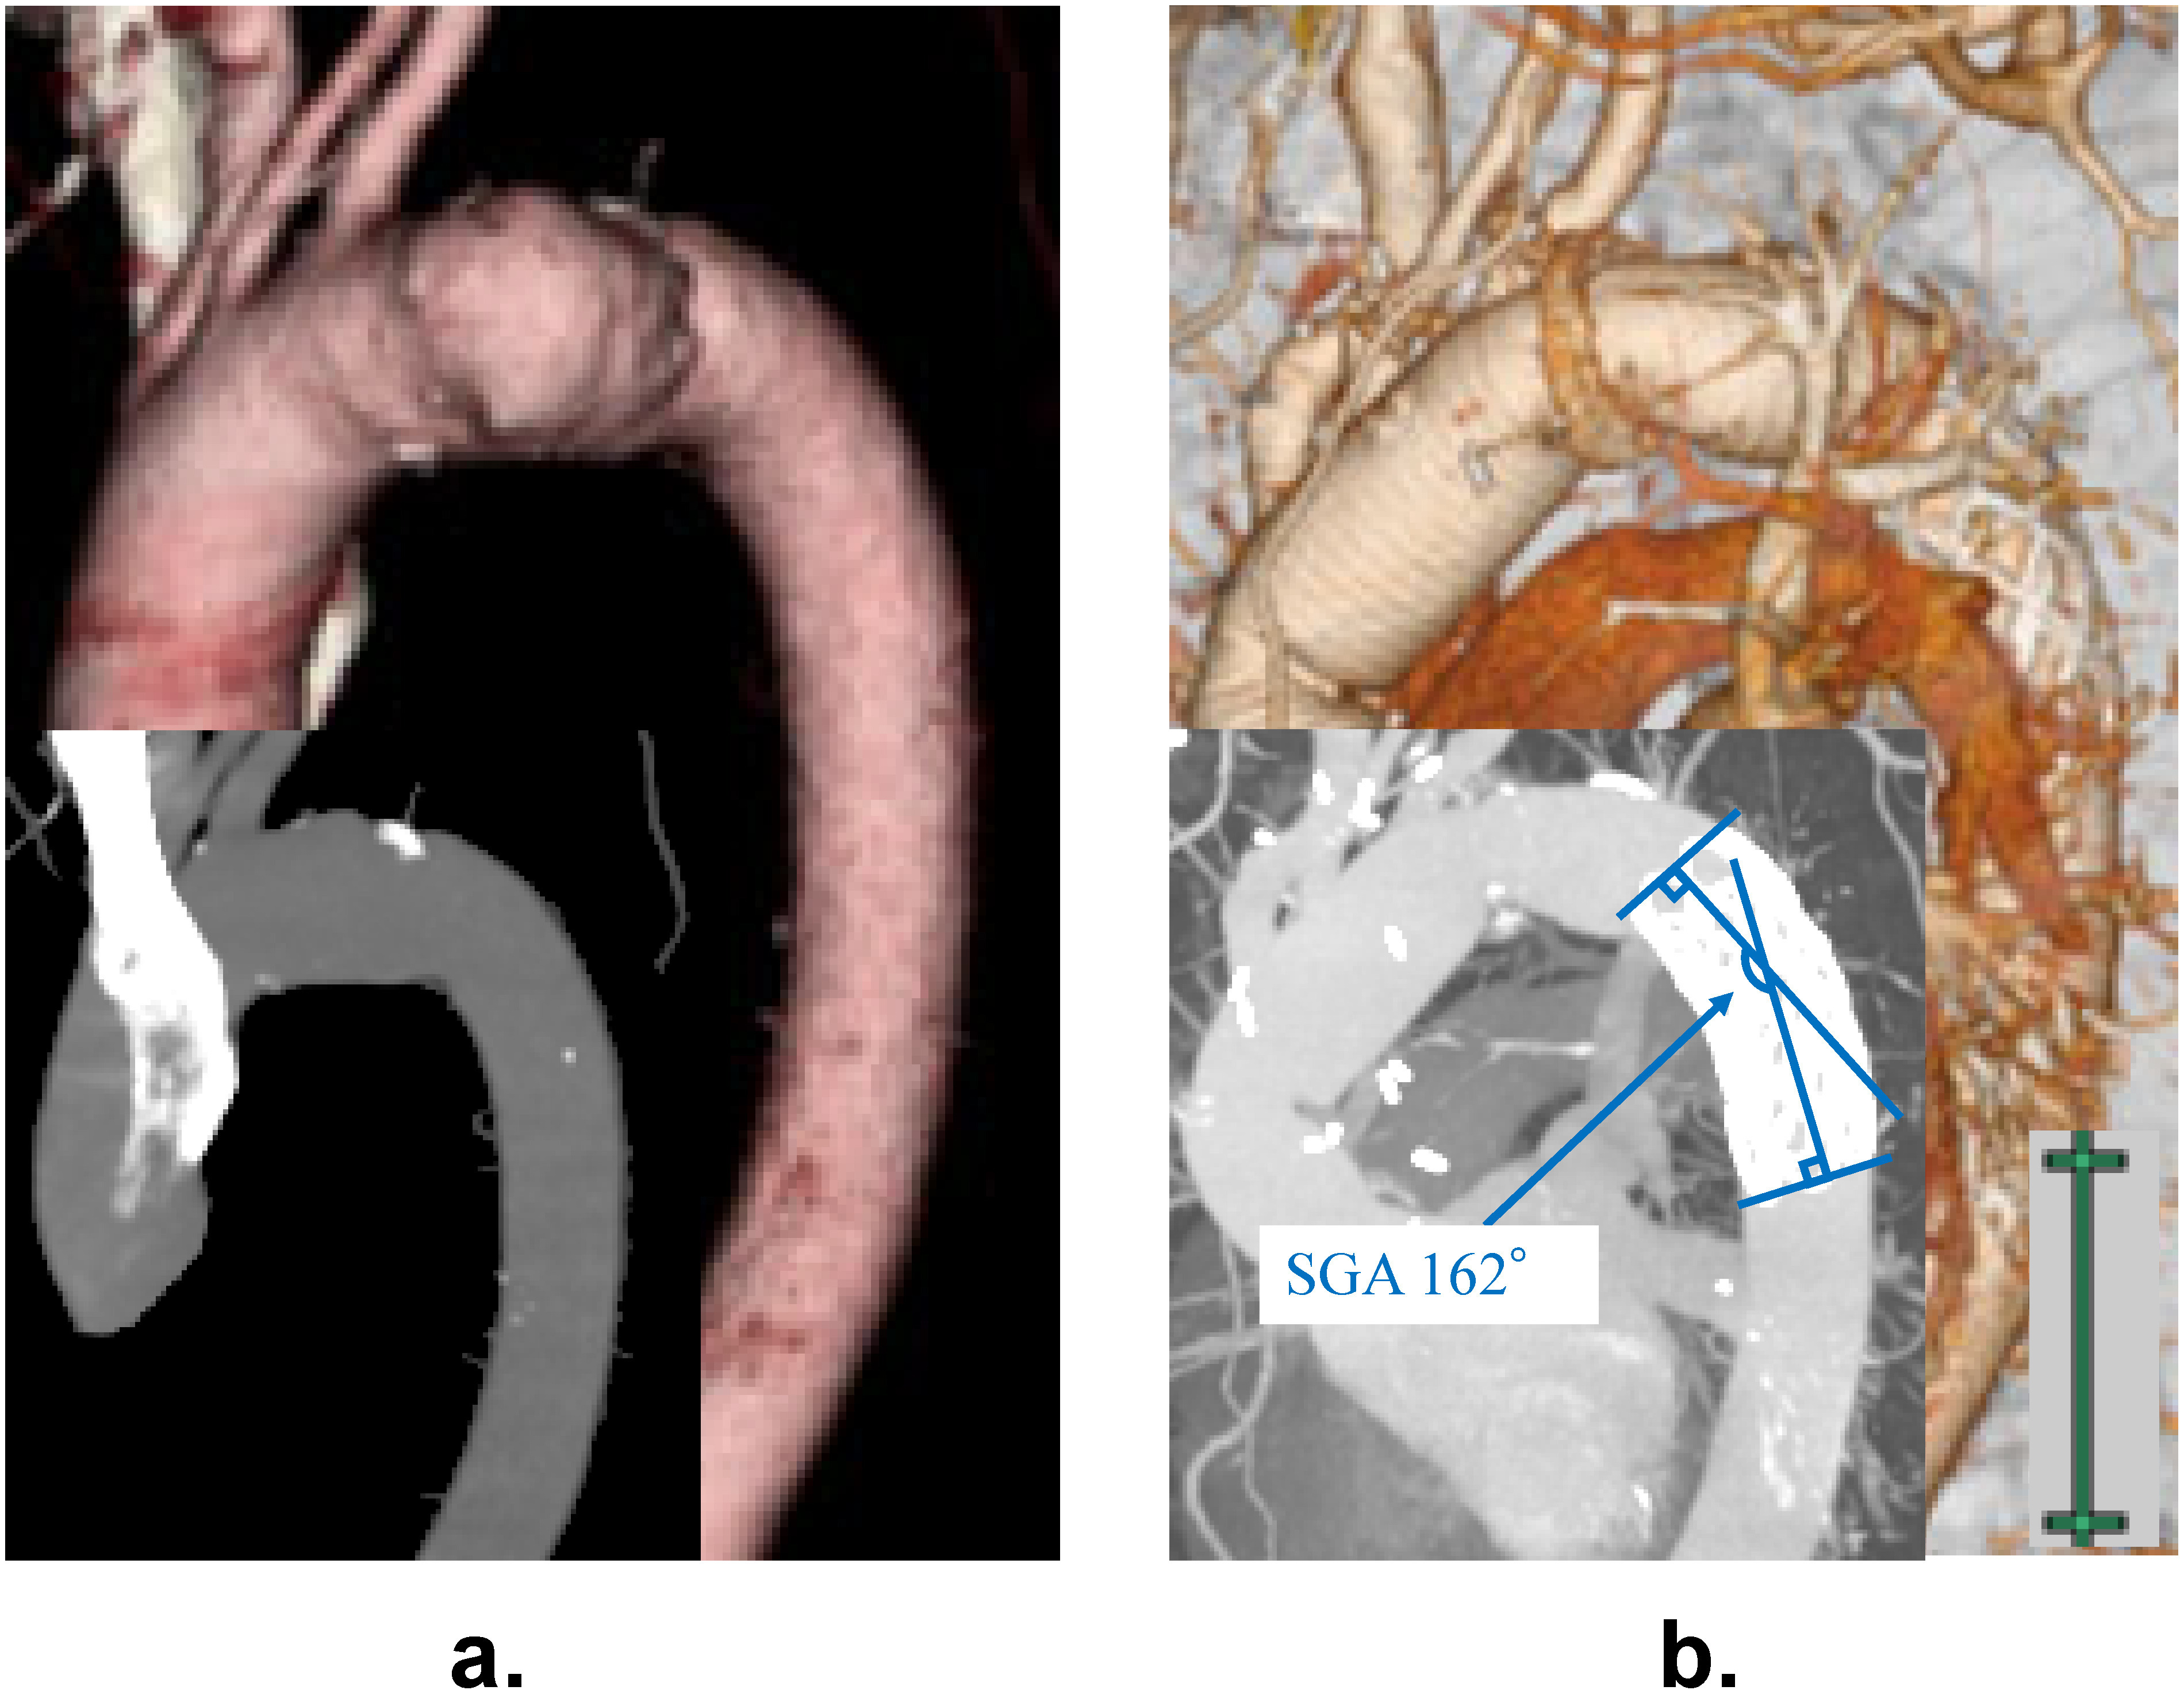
Fig. 1 Pre- and post-operative CT images with three-dimensional reconstruction before and after the total arch replacement using the frozen elephant trunk technique. (a) Preoperative CT image showing a saccular-type aortic arch aneurysm. The size of the descending thoracic aorta was 26.5 mm at Th6 and gradually tapered to 24.4 mm at Th8. (b) Postoperative CT image showing the total arch replacement using the frozen elephant trunk procedure with 27-mm JOSG. The SGA was 162° at this time.
